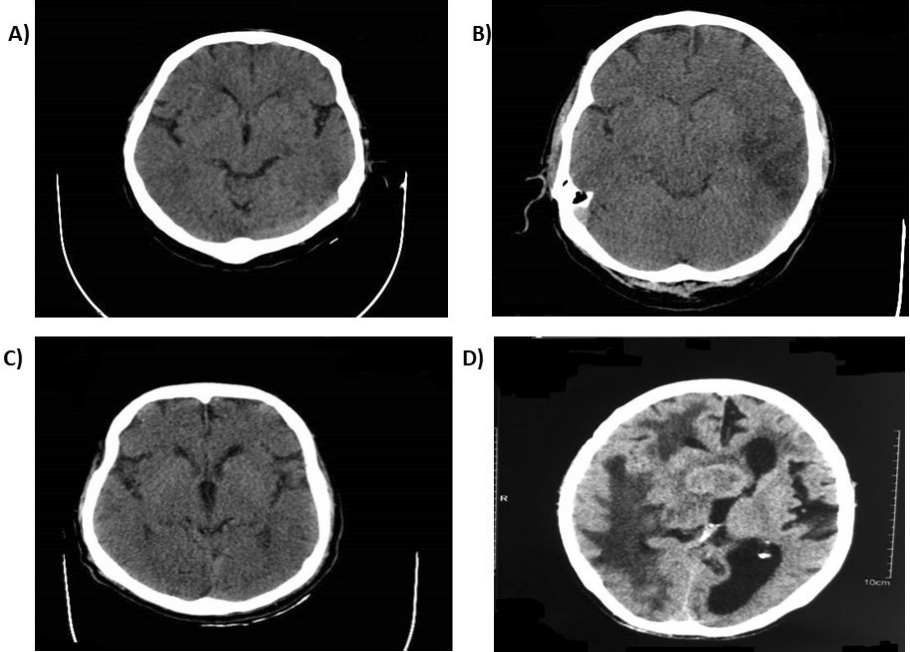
CT-scan of the patients with the extreme alleles. (A) 17-repeat, (B) 17-repeat, (C) 42-repeat, (D) 43-repeat. Extensive hypodense areas and calcification were detected in various brain sections.
